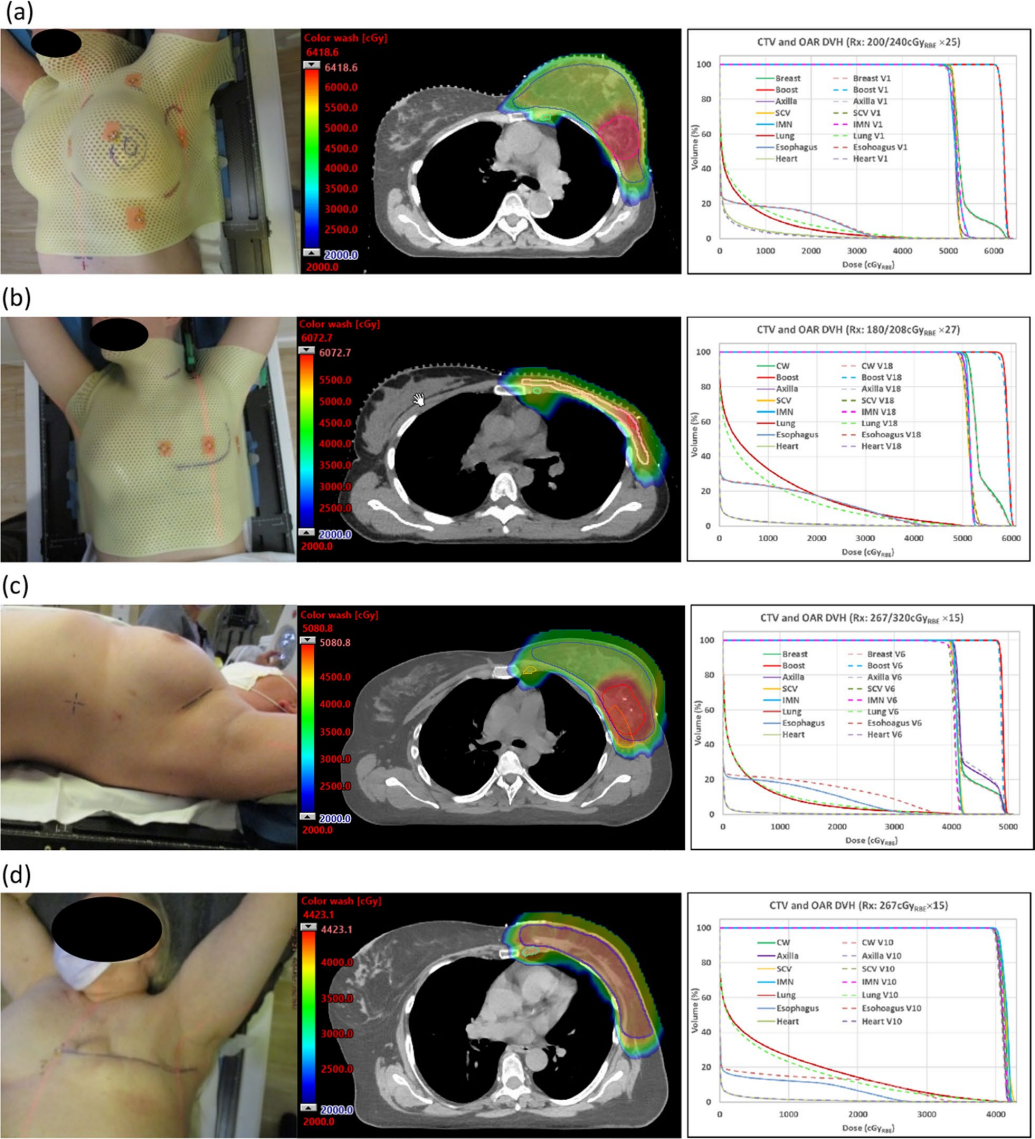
Patient setup, treatment plan dose and DVH for a a masked breast patient, b a masked CW patient, c a maskless breast patient, and d a maskless CW patient. Abbreviations: CTV, clinical target volume; OAR, organ at risk; DVH, dose volume histogram; Rx, prescription; Gy_RBE_, Relative-Biological-Effectiveness dose in Gray; CW, chest wall; Vx, verification Computed Tomography at fraction x; SCV, supraclavicular node; IMN, internal mammary nodes
